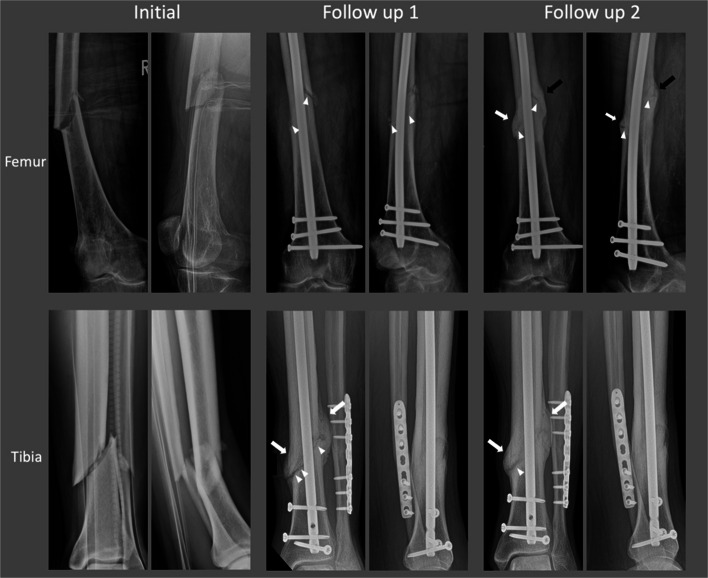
Examples of femur (top) and tibia (bottom) long bone fractures in X-rays with their corresponding follow-up images during healing (from left to right). Top: Femoral shaft fracture treated using nail fixation. No callus is seen at first follow-up image and the fracture line is still visible (white arrowhead). At the second follow-up, a callus bridging is seen at all cortices, however the dorsal and medial callus (black arrow) appears larger than lateral and ventral (white arrows). Bottom: tibial shaft fracture and fracture fixation with nailing and fibular plate. Callus (white arrows) and fracture line (white arrowhead) is seen in the first follow-up. At the second follow-up, the callus appears denser compared to its follow-up and the fracture line remains visible
